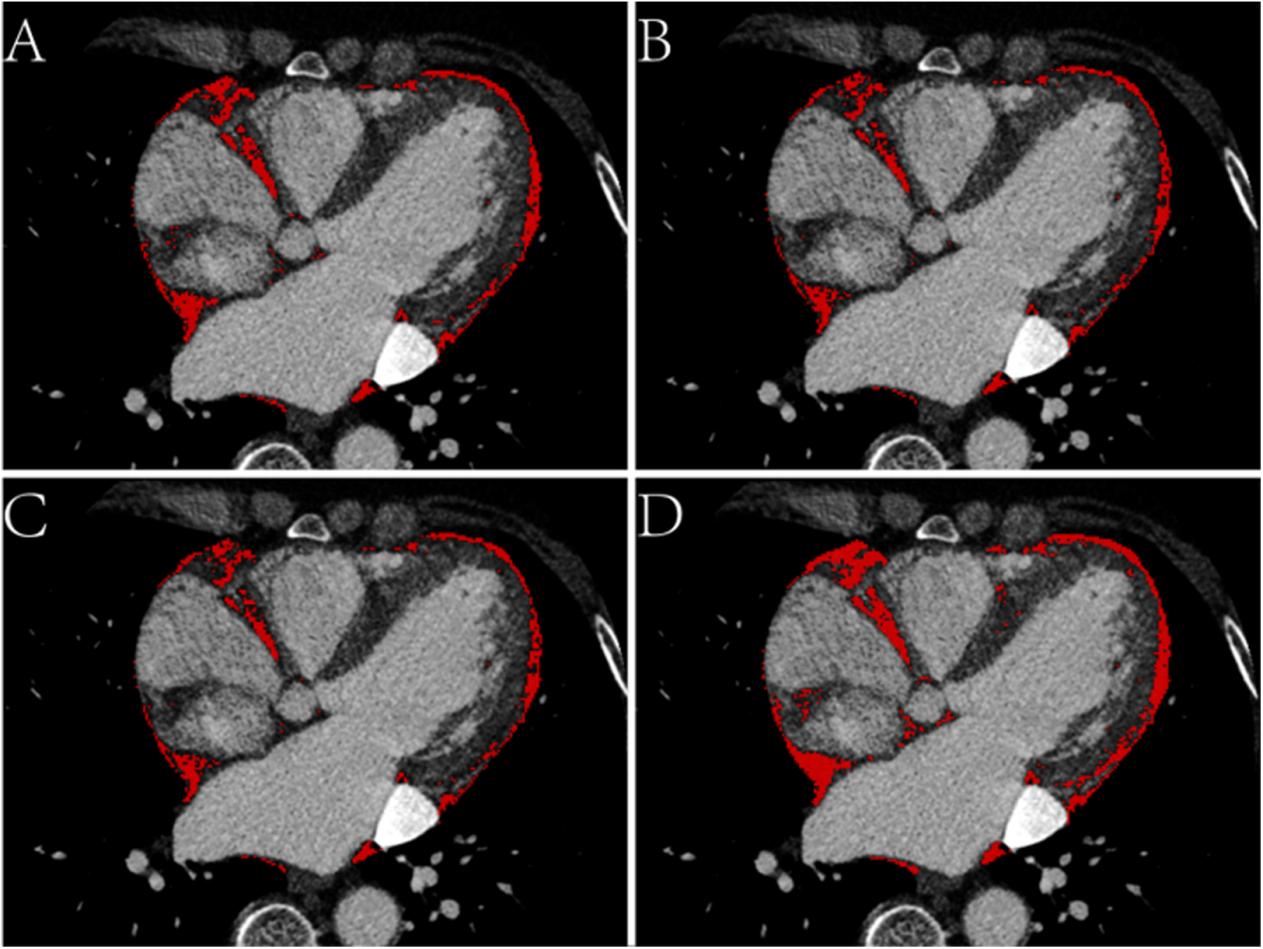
Axial CT images of the epicardial adipose tissue (EAT) based on four attenuation ranges. (A) The red area represents the EAT based on -190 to -30 HU. (B) The red area represents the EAT based on -195 to -45 HU. (C) The red area represents the EAT based on -200 to -45 HU. (D) The red area represents the EAT based on -200 to 0 HU.
